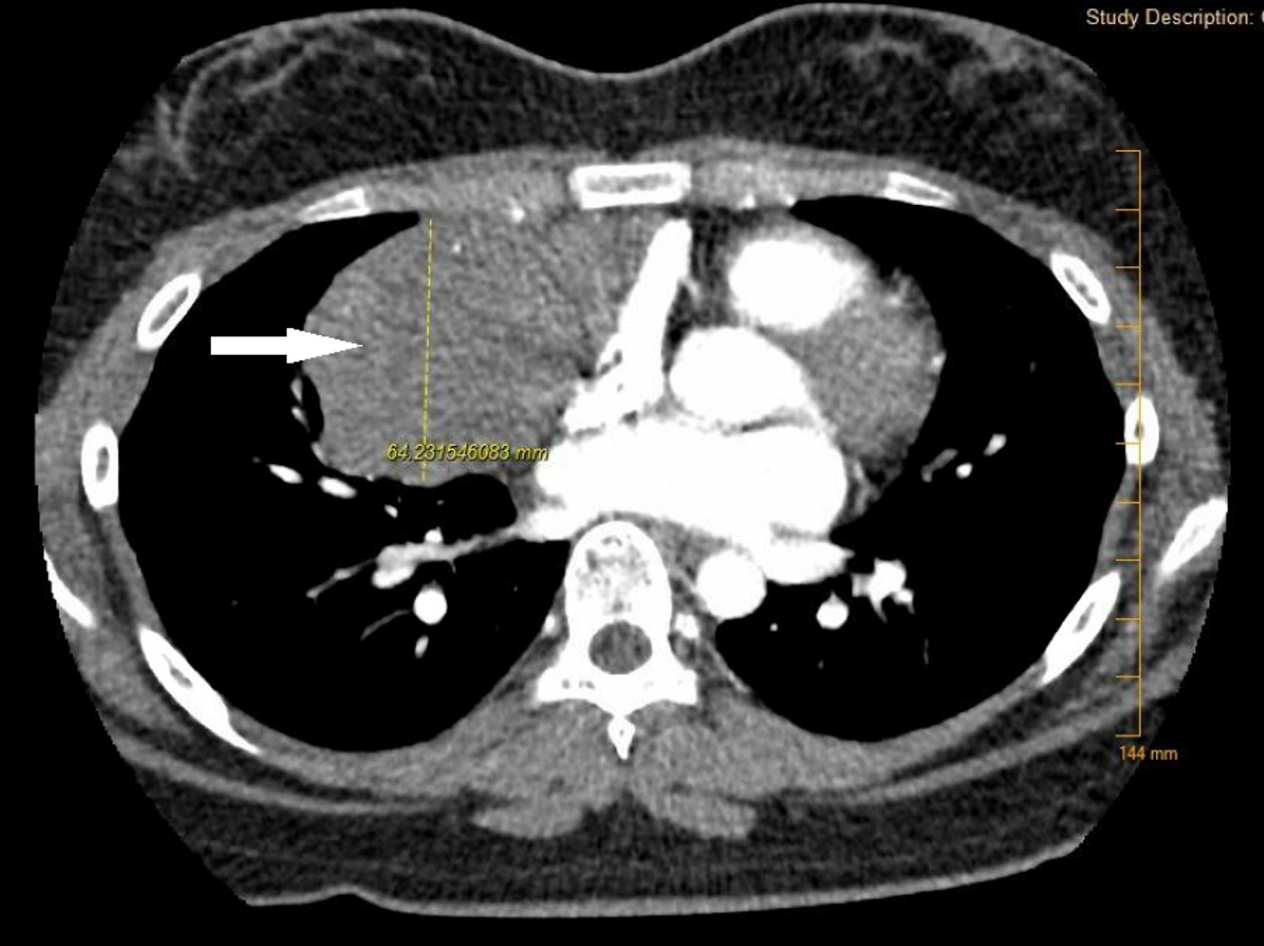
Axial view computed tomography scan of chest with contrast demonstrating 10.7 cm x 4 cm x 10.1 cm mass (white arrow) inseparable from the right heart border pericardium, displacing the heart to the left with associated compression of the superior right atrium and the superior vena cava
